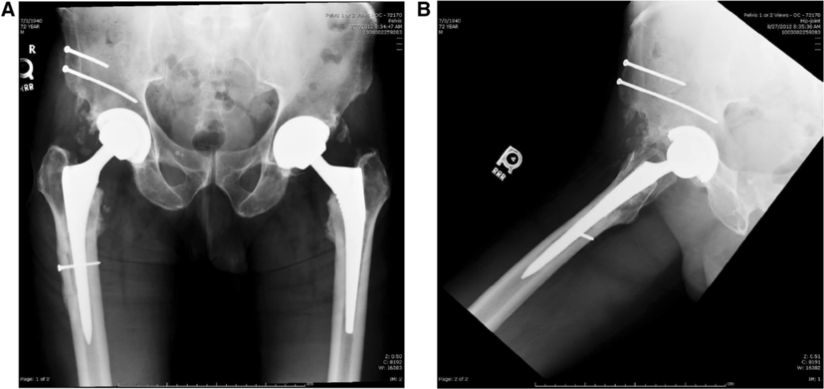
Post-operative anteroposterior (AP) and lateral hip radiographs (A-B) demonstrating the revision femoral stem/head, repair of the iliac osteotomy using two 3.5 mm cortical screws, and repair of the femoral corticotomy with a 3.5 mm cortical lag screw.
